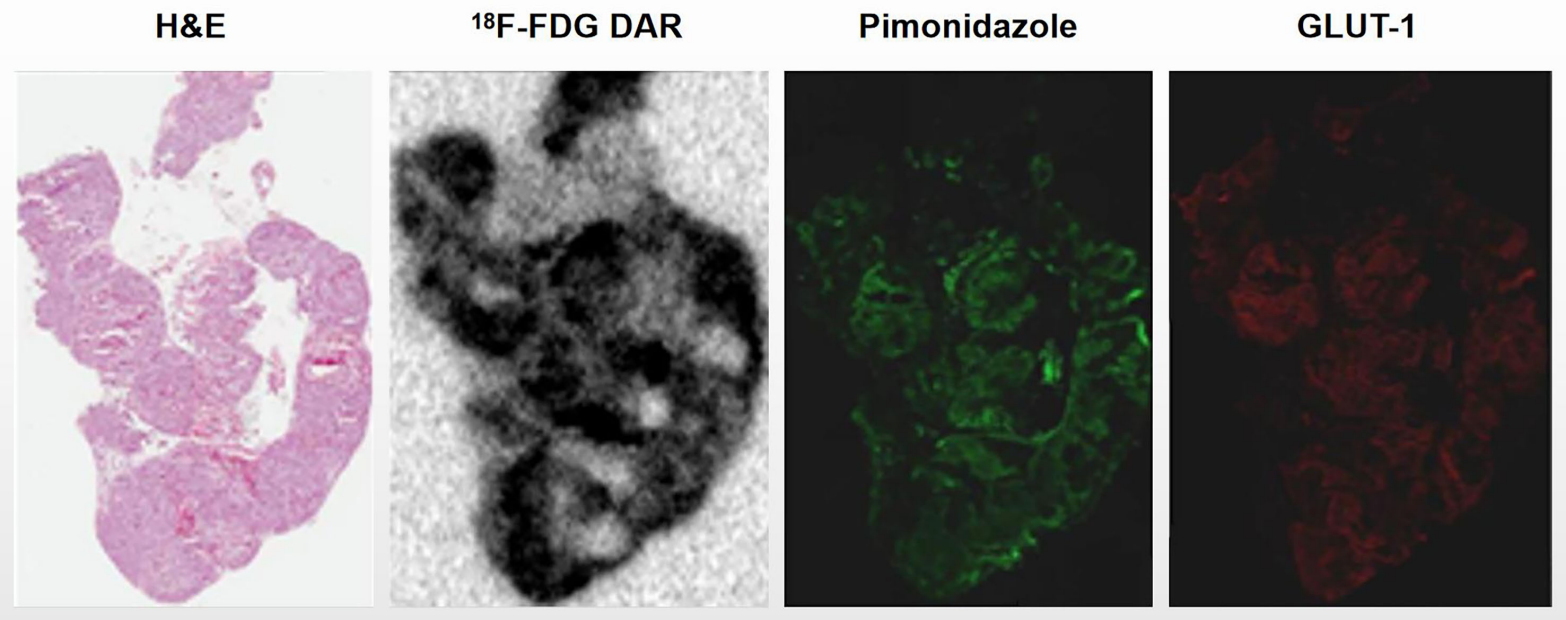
Ascites tumors showed high ^18^F-FDG accumulation. Ascites tumor cells of HTB177 cell line. Tumor cells showed high uptake of ^18^F-FDG by DAR, suggesting that their glucose metabolism activity was increased; Meanwhile, hypoxia markers pimonidazole and GLUT-1 were also highly expressed.
